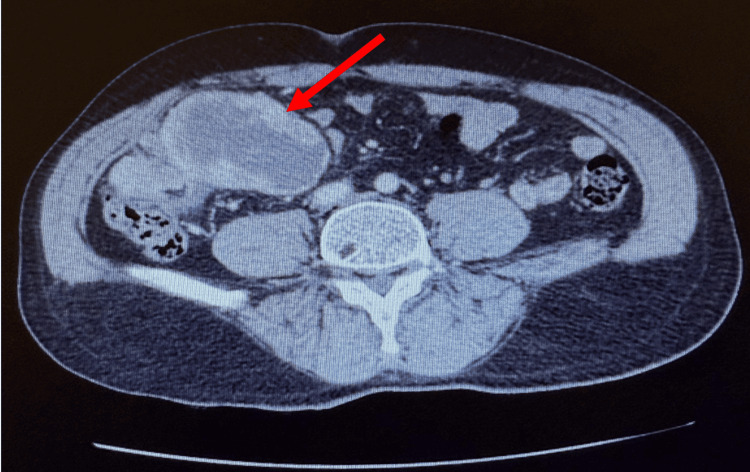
Axial contrast-enhanced CT scan of the abdomen. A large heterogeneous mass in the right iliac fossa (red arrow), without evidence of locoregional invasion and with preserved surrounding fat planes. The lesion measured approximately 105 × 70 × 100 mm and appeared solid, irregular, and well circumscribed.
